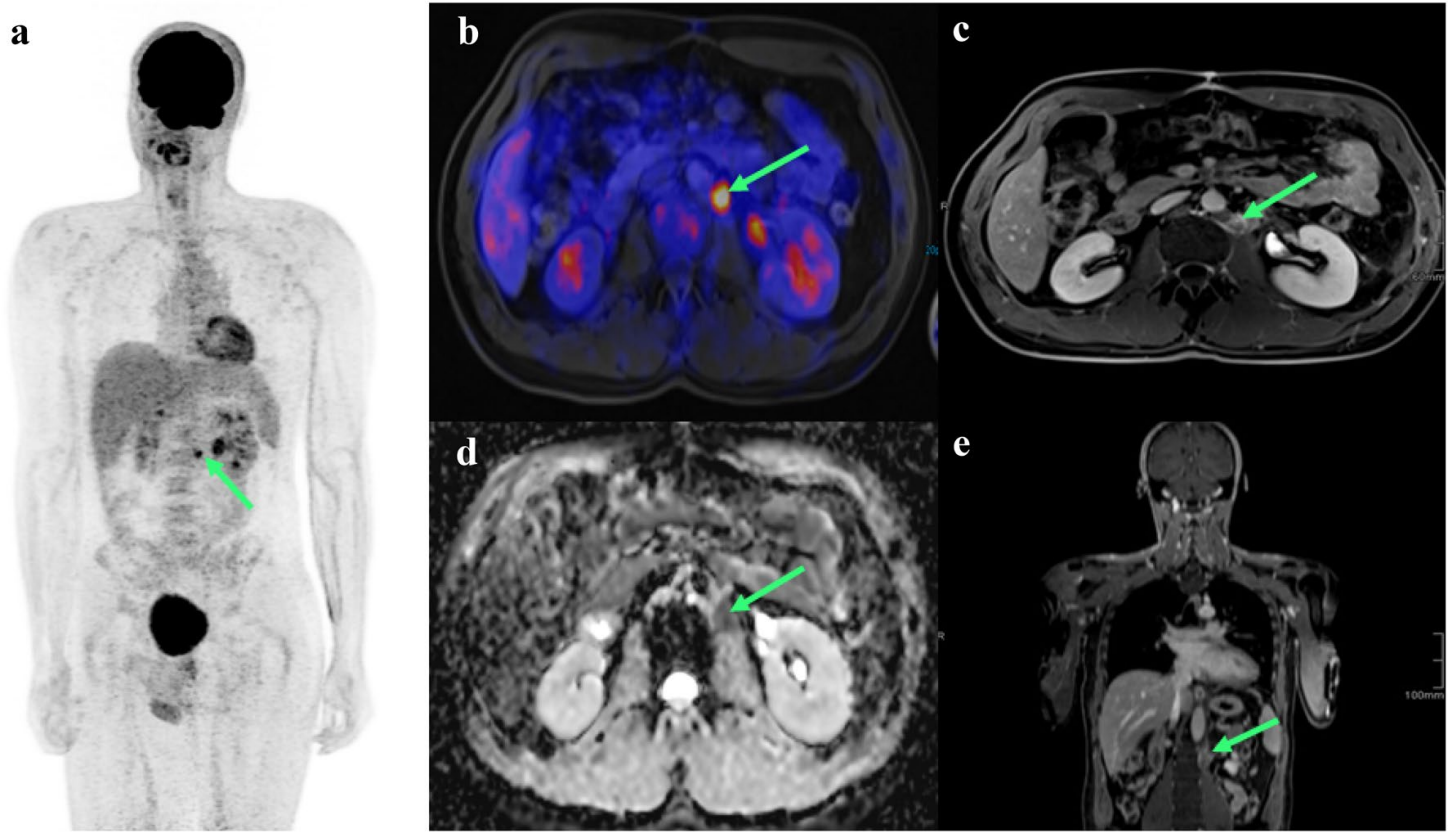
Abdominal FDG-PET/MRI of a retroperitoneal residual tumor in metastatic seminoma after chemotherapy. A: Inverted maximum intensity projection (MIP) of an whole-body PET/MRI with a paraaortal lymph node (green arrow). B: Transversal image of the abdominal PET/MRI in fusion sequence with high FDG uptake due to increased metabolism (green arrow). C: Transversal image of the abdominal PET/MRI in T1 sequence with hyperintens lymph node after contrast agent administration (green arrow). D: Transversal image of the abdominal PET/MRI in DWI sequence with hypointens lymph node (green arrow). E: Coronar image in T1 sequence with hyperintens lymph node after contrast
